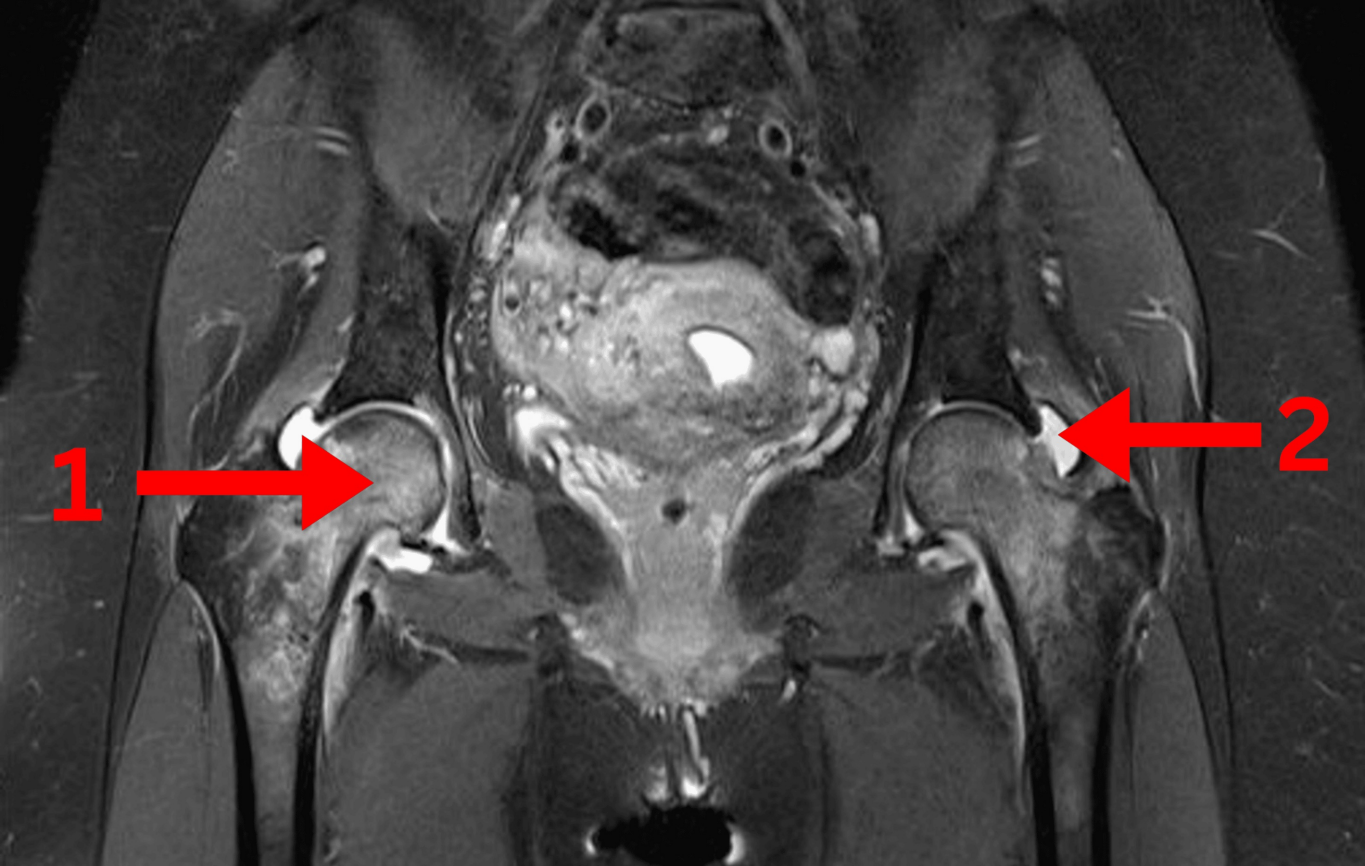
MRI of the hips without contrast showing (1) STIR bright signals and (2) hip joint effusion MRI, magnetic resonance imaging; STIR, short tau inversion recovery.
